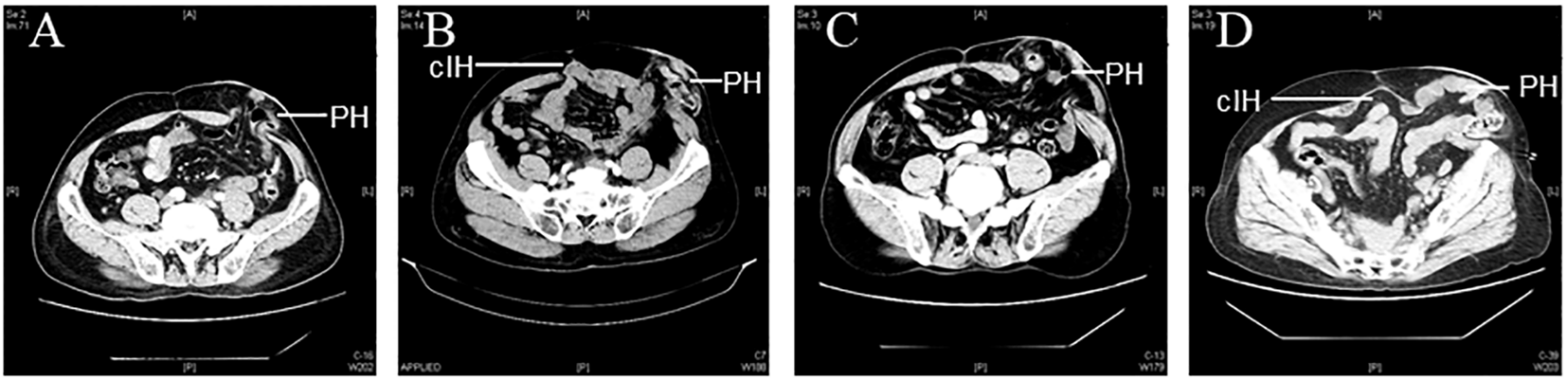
Representative CT images of parastomal hernia. (PH: parastomal hernias; cIH: concomitant incisional hernias) (A) Type I: PH≤ 5 cm without cIH; (B) Type II: PH ≤5 cm with cIH; (C) Type III: PH>5 cm without cIH; (D) Type IV: PH>5 cm with cIH.
